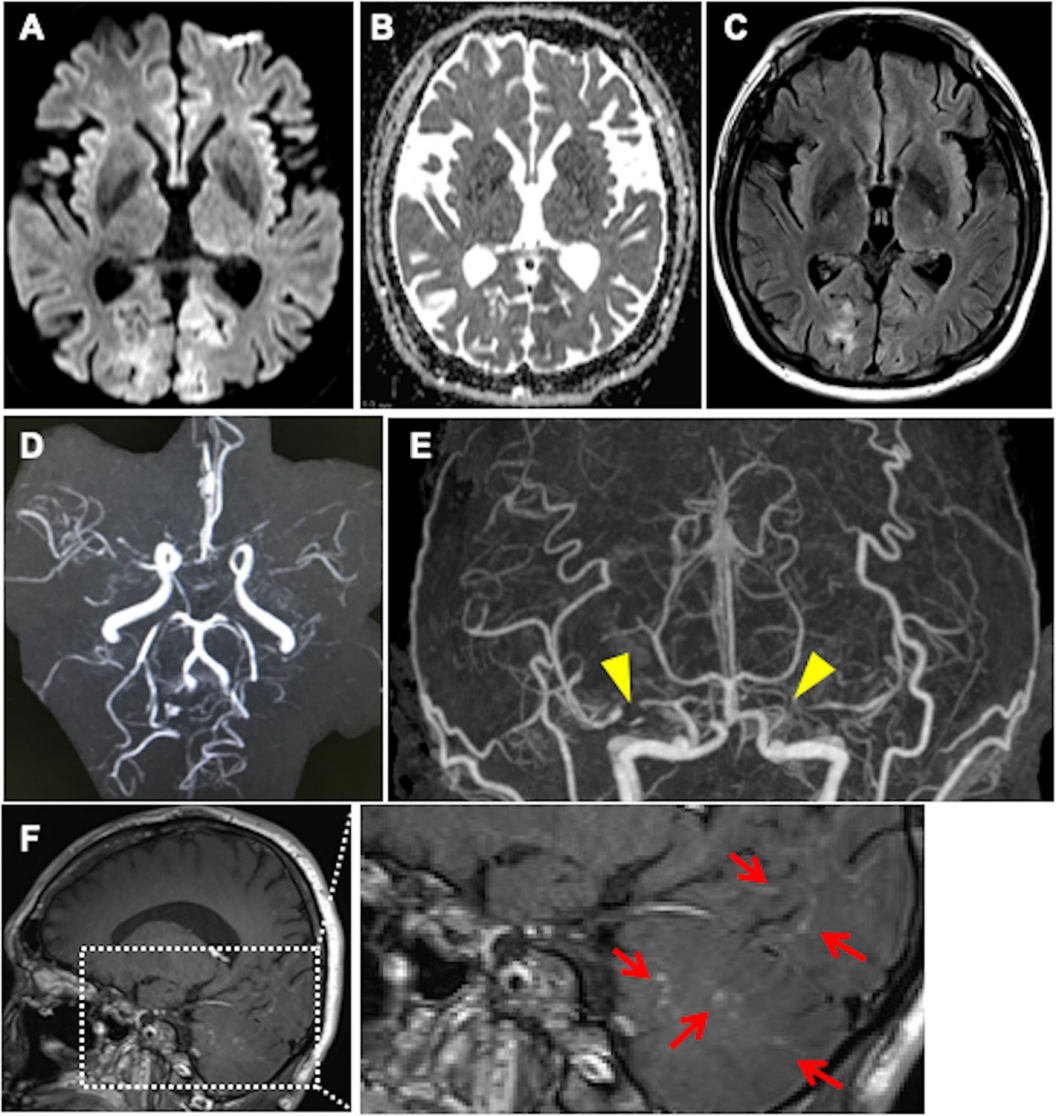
Radiological observations on the second admission a–c. Brain magnetic resonance imaging (MRI) on the second admission shows hyperintense lesions in the bilateral occipital lobe on diffusion-weighted imaging (a), apparent diffusion coefficient map (b), and fluid-attenuated inversion recovery (c). d. MR angiography shows stenosis in bilateral middle cerebral arteries (MCAs). e. Three-dimensional contrast-enhanced angiography revealed occlusions in bilateral MCAs (yellow arrowheads). f. Contrast-enhanced MRI shows multiple punctate and linear gadolinium-enhanced lesions (red arrows) in the occipital and temporal lobes and cerebellum
